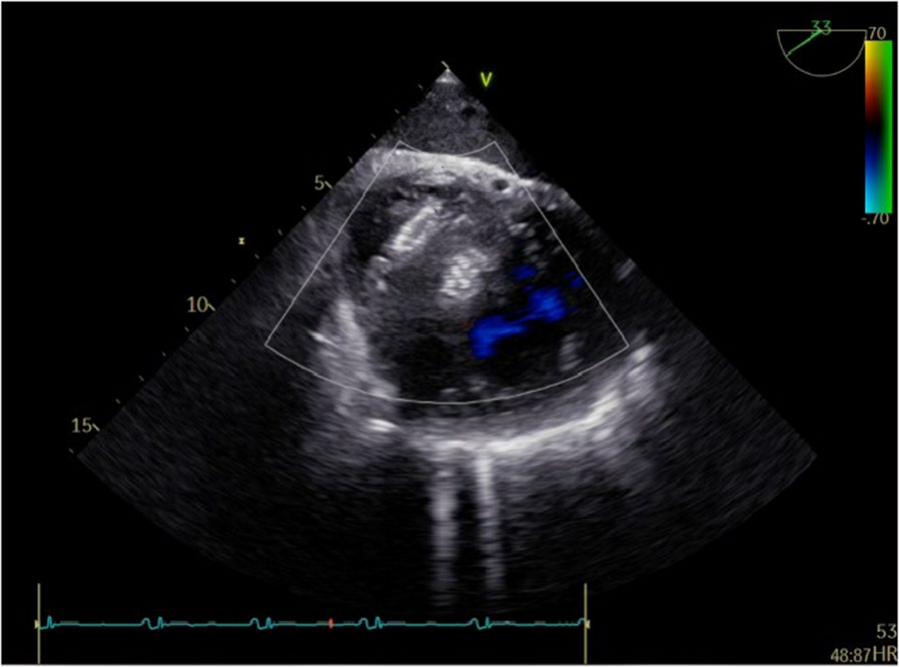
Transoesophageal echocardiography (TEE) post-procedural showed a 20-mm ASD occluder device (Ref FQFDQ-I 20, SN 24031223, Lot 202,403) was successfully placed into VSR area, with minimal residual flow
